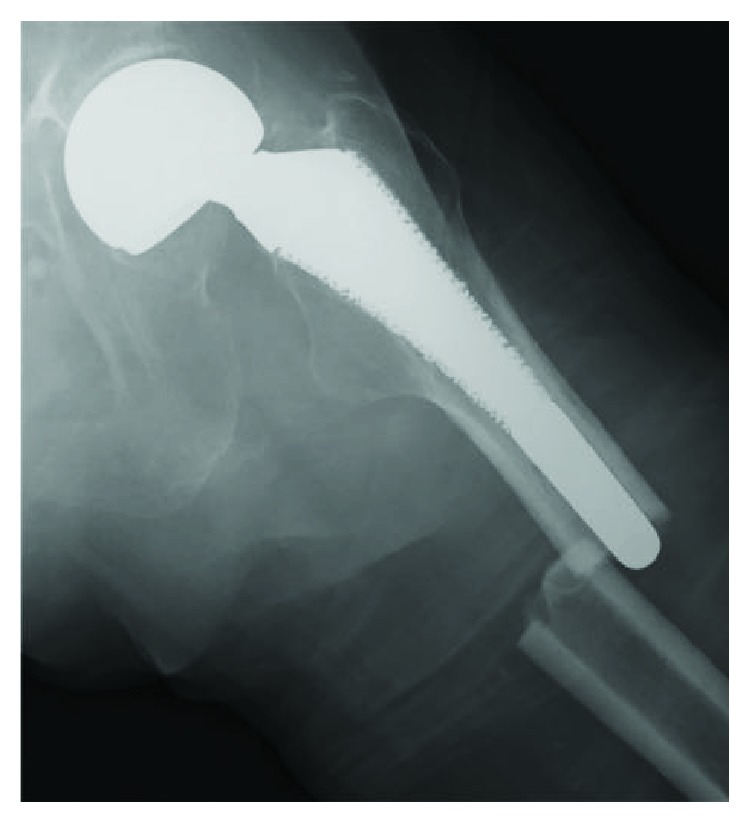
Anteroposterior pelvis radiograph showing a noncomminuted transverse fracture located at the tip of the stem with localized periosteal thickening of the lateral cortex, or a “beak sign,” of the left femur.
